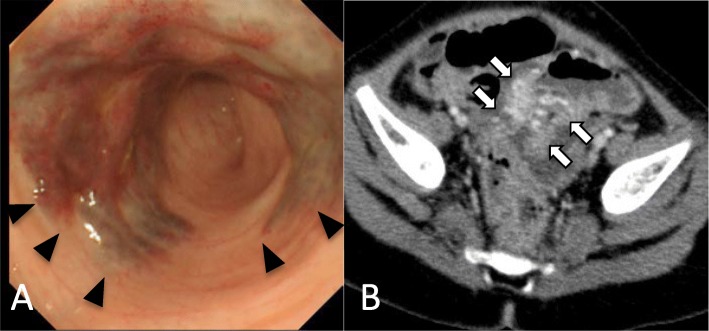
Imaging of the arteriovenous malformation. a Colonoscopic view showing the protruding lesion, which was suggestive of a submucosal vascular abnormality. b Contrast-enhanced computed tomography image showing dilation of the blood vessels in the lesion, but no hypertrophied feeding artery or draining vein
